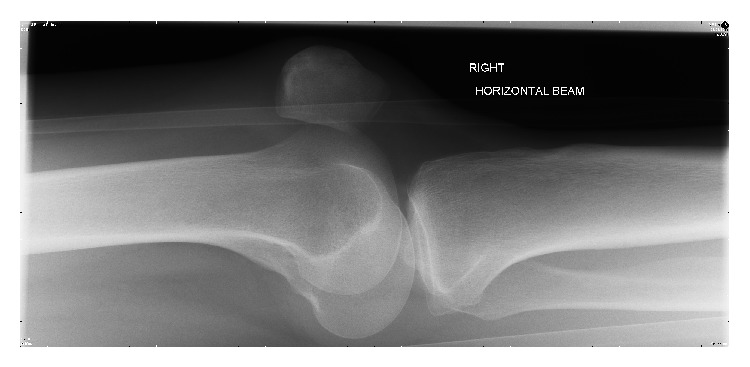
Lateral radiograph of the knee. The knee is held in extension and the patella is rotated 90 degrees.
